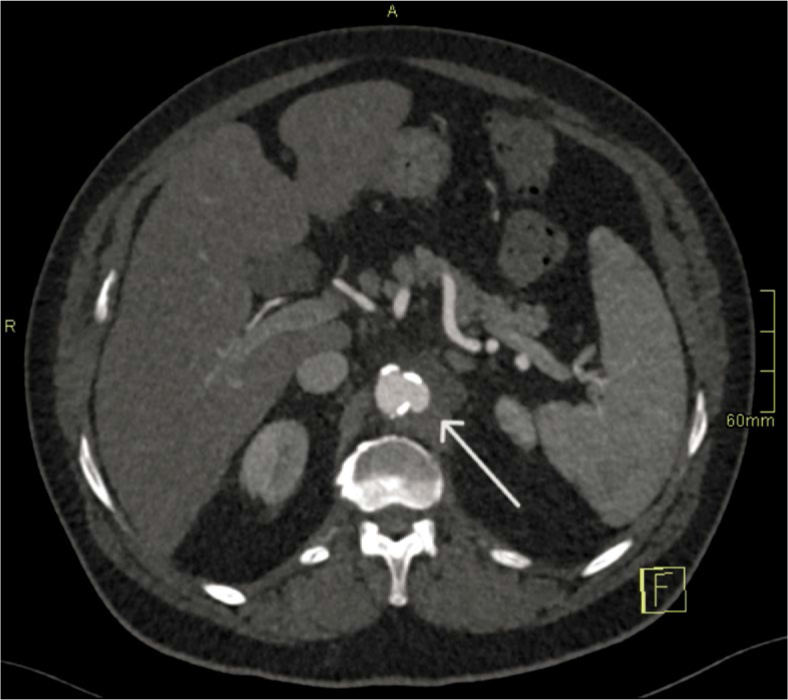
Hyper-rapid progression (Day 6–7). Axial CT angiography (CTA) demonstrates rapid expansion of the pseudoaneurysm to 13 × 7 mm over 72 hours (arrow).
